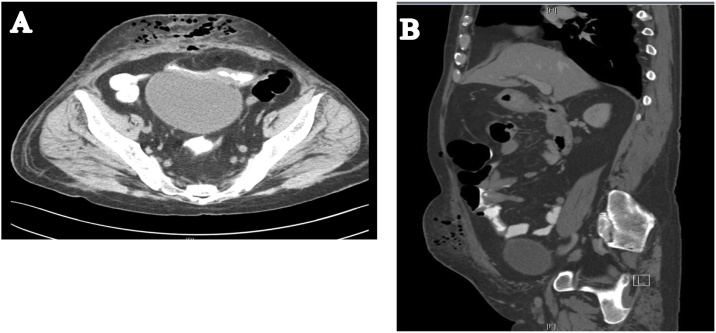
A and B, Abdomen CT with IV and oral contrast showed thicking of terminal ileum.
